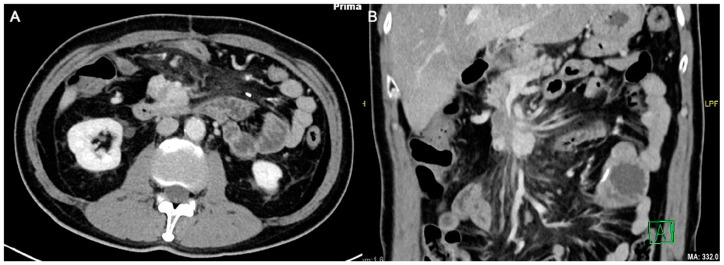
Axial (A) and coronal (B) computed tomography images 1 year post open irreversible electroporation. The pancreatic mass now measures approximately 3.9 × 2.6 cm.
